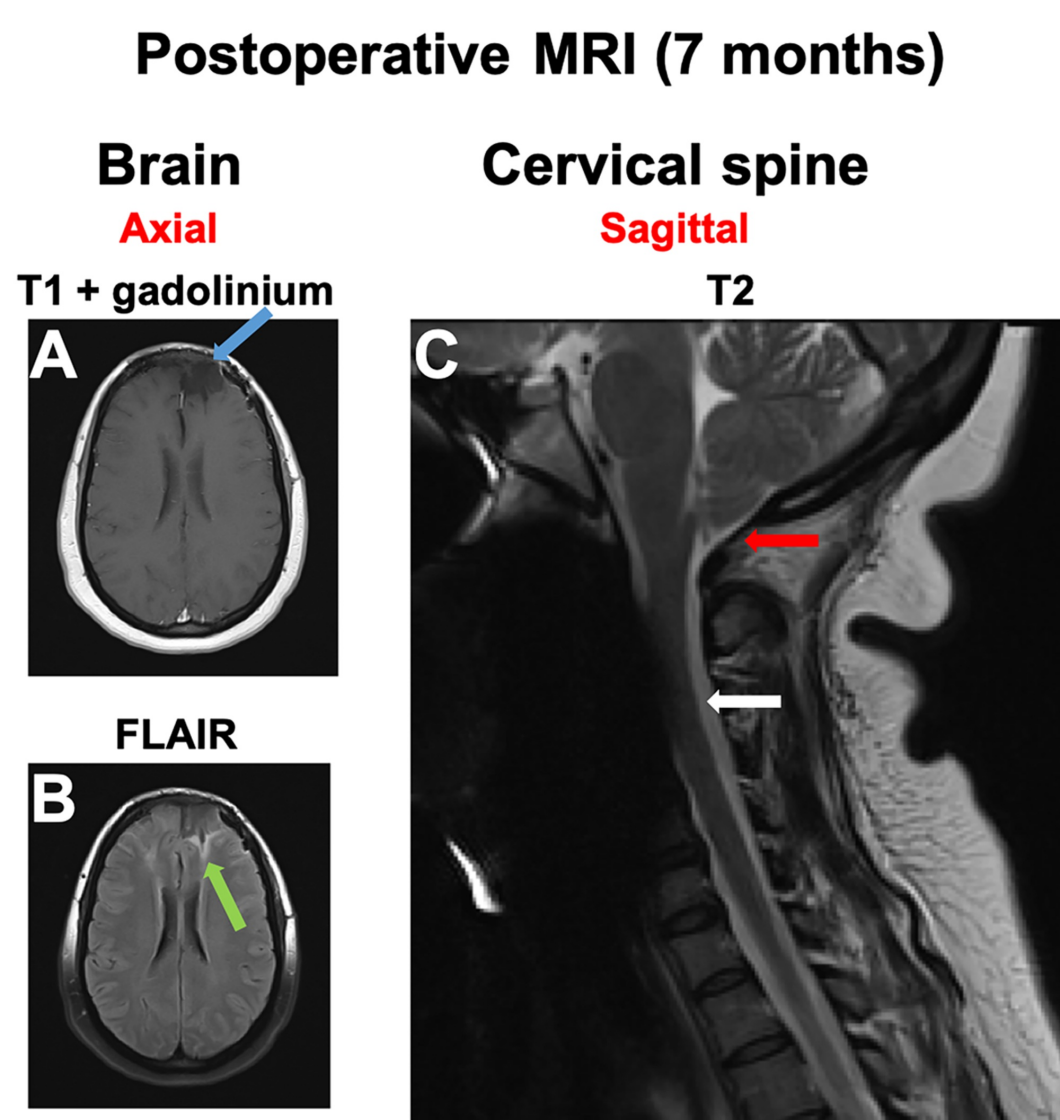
Postoperative MRI imaging A,B. At seven months after the procedure, MRI of the brain (left) showed (A) no tumor recurrence (blue arrow on T1 + gadolinium image) and (B) near-complete resolution of the peritumoral edema (green arrow on FLAIR image). C. The cervical spine MRI demonstrated resolution of the tonsillar herniation (red arrow) and cervical syrinx (white arrow). FLAIR: fluid-attenuated inversion recovery
